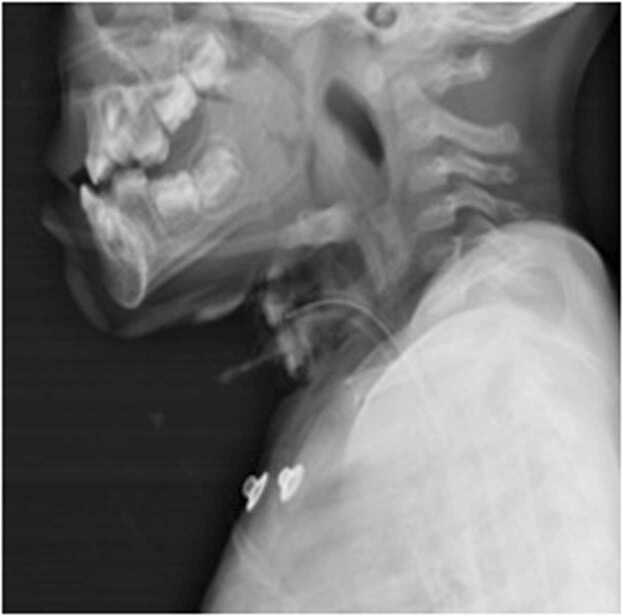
Post-operative lateral neck x-ray – remarkable reduction of soft tissue widening noted in comparison to pre-operative x-ray (Fig. 3), with Tracheostomy tube in position.
